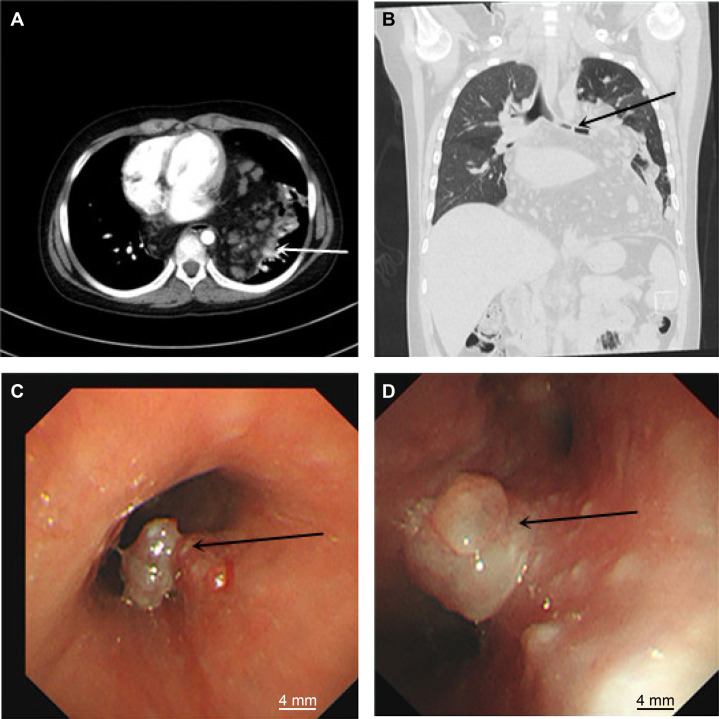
(A) Axial CT scan of the chest showing the tumour encasing the mediastinal structures and protruding into both lung fields (white arrow). (B) Coronal CT scan of the chest showing the tumour involving the left lung and bronchus along the vascular spaces (black arrow). (C) Bronchoscopy showing a tumour obstructing the airway at the left main bronchus and carina between the left upper and lower lobes (black arrow). (D) Bronchoscopy showing a tumour obstructing the airway at the left upper and lower lobes (black arrow).
